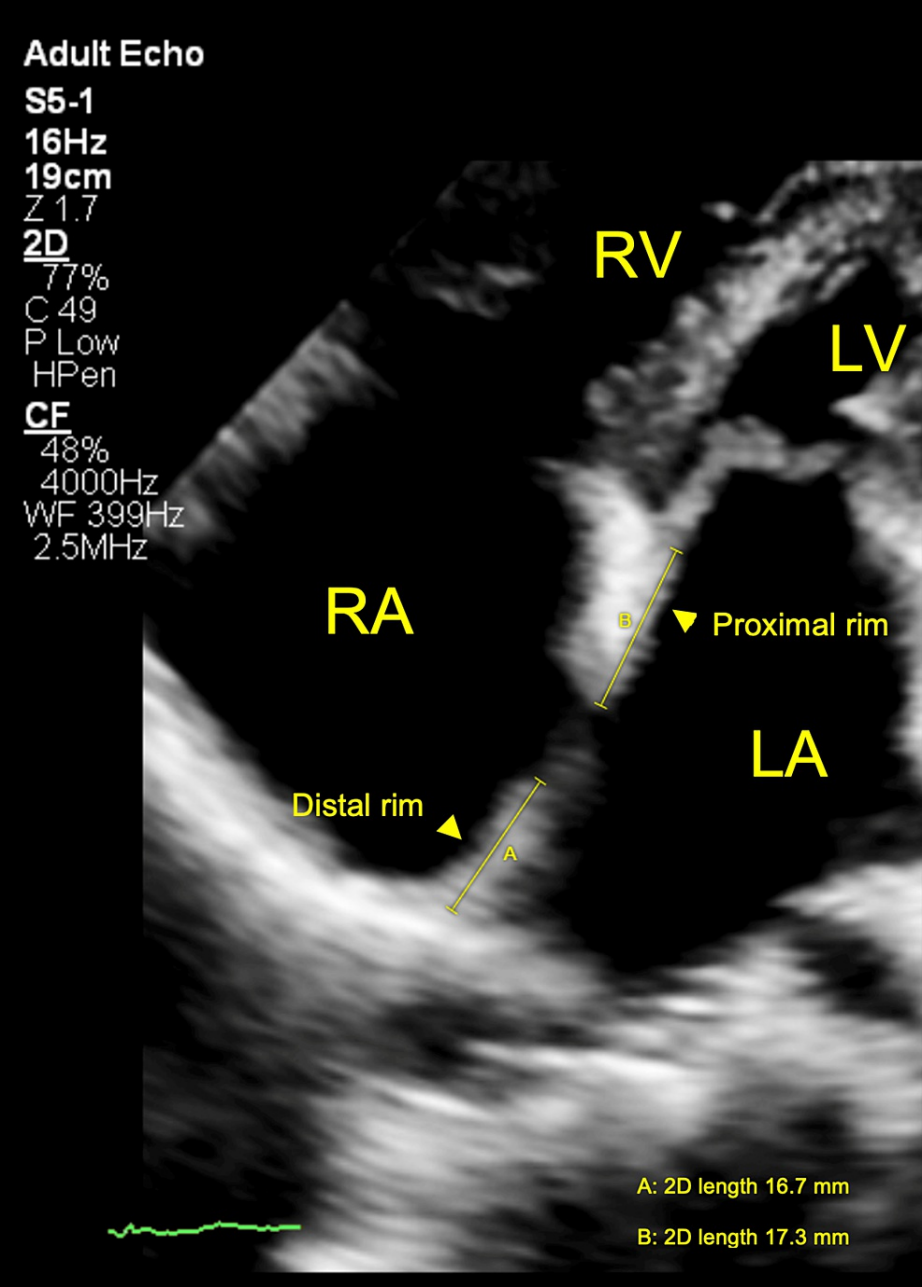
Two-dimensional TTE, apical four-chamber view, systolic phase, demonstrates a large ASD with both proximal (distance B) and distal (distance A) rims measuring approximately 17 mm each. TTE: Transthoracic echocardiography; ASD: Atrial septal defect.
